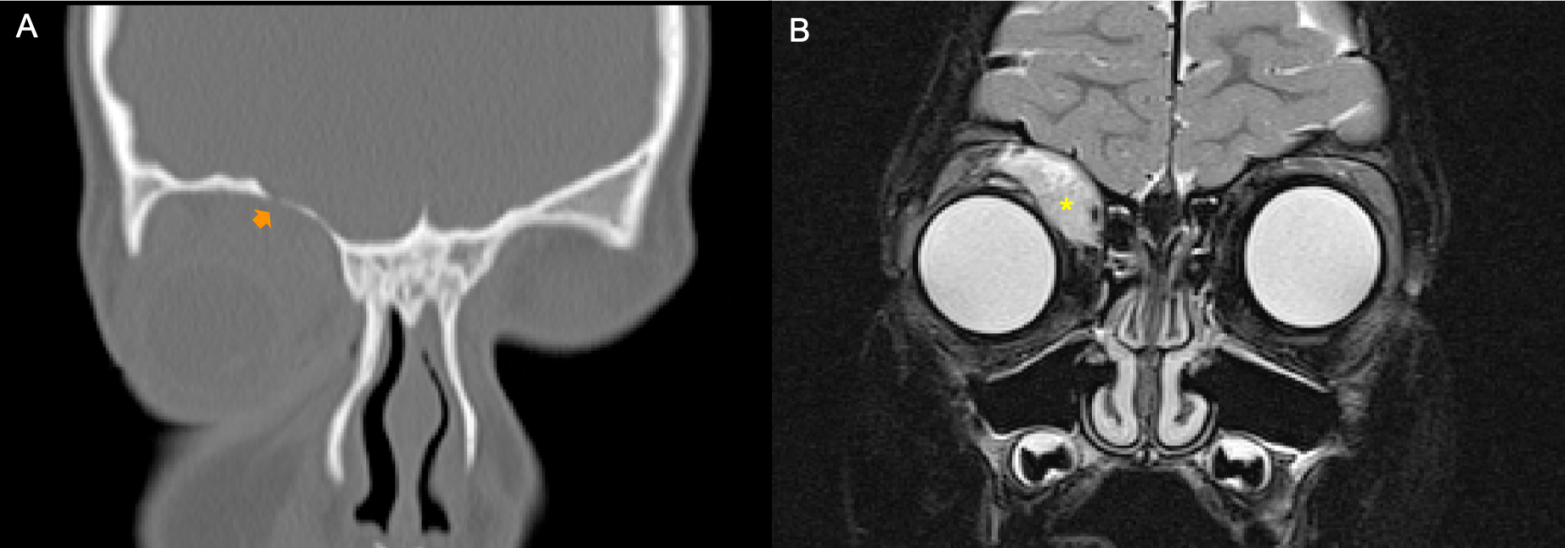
Example of a patient with orbital rhabdomyosarcoma (RMS) and moderate bone erosion (BE). CT scan and MRI were performed at staging. (A) Bone window coronal reconstruction of the CT scan showed moderate grade of BE (*orange arrow*). (B) Coronal fat-saturated T2-weighted image of magnetic resonance well demonstrated a right orbital tumor (*yellow asterisk*).
